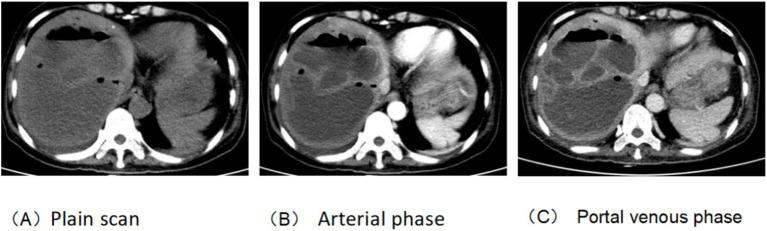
Upper abdominal enhanced CT. (A) Plain scan, large low-density shadows in the right and left inner lobes of the liver, Size about 14x10cm; there is separation and gas accumulation inside; (B,C) the lesion wall and septum were enhanced, which was considered liver abscess.
